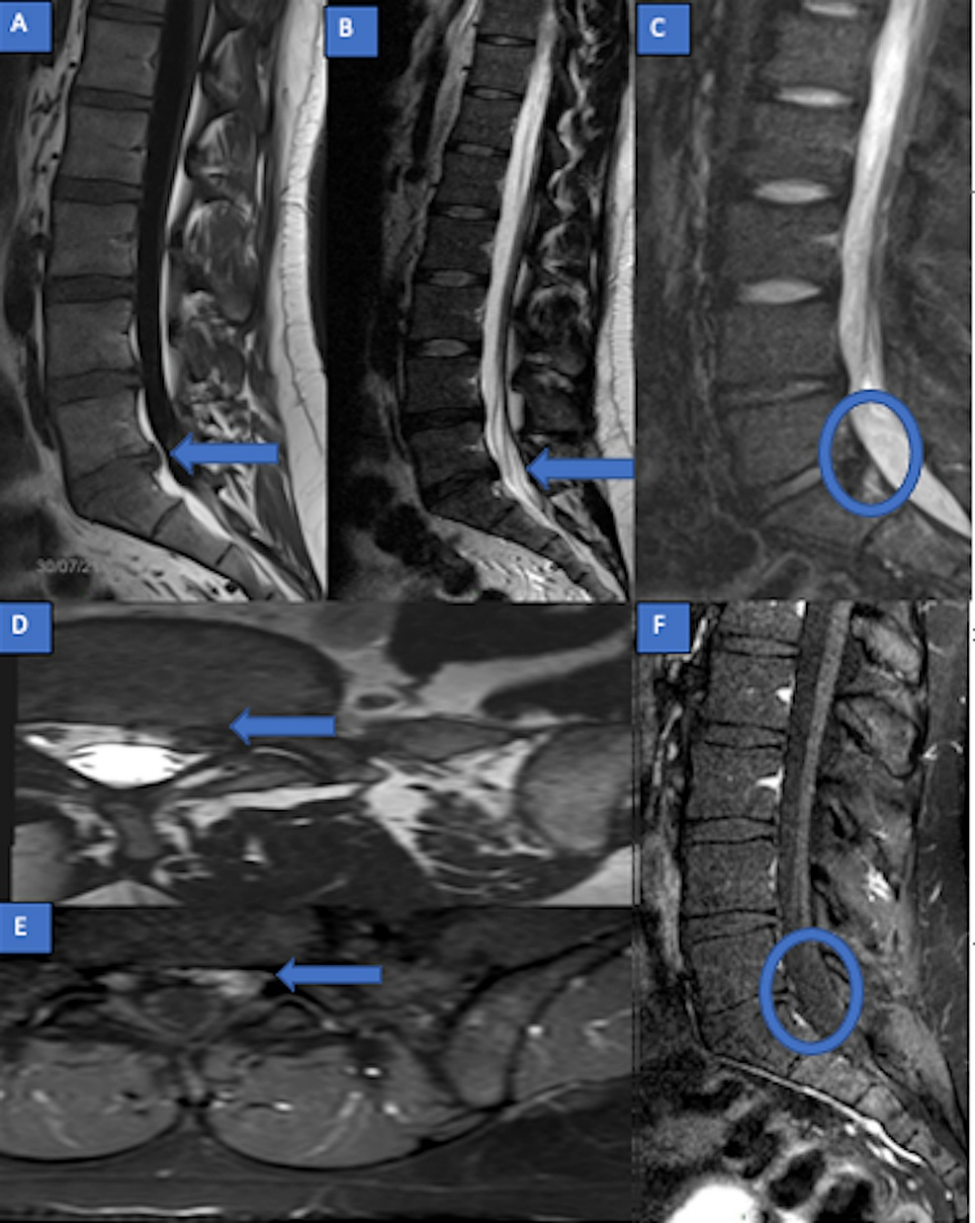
Lumbar spine MRI (February 2021) (A) Sagittal T1, (B) T2, (C) T2 STIR; (D) axial T2; (E, F): axial and sagittal T1 post-contrast: posterior descending discal protrusion, in conflict with the left S1 root. There is no intracanalar mass. MRI - Magnetic Resonance Imaging
